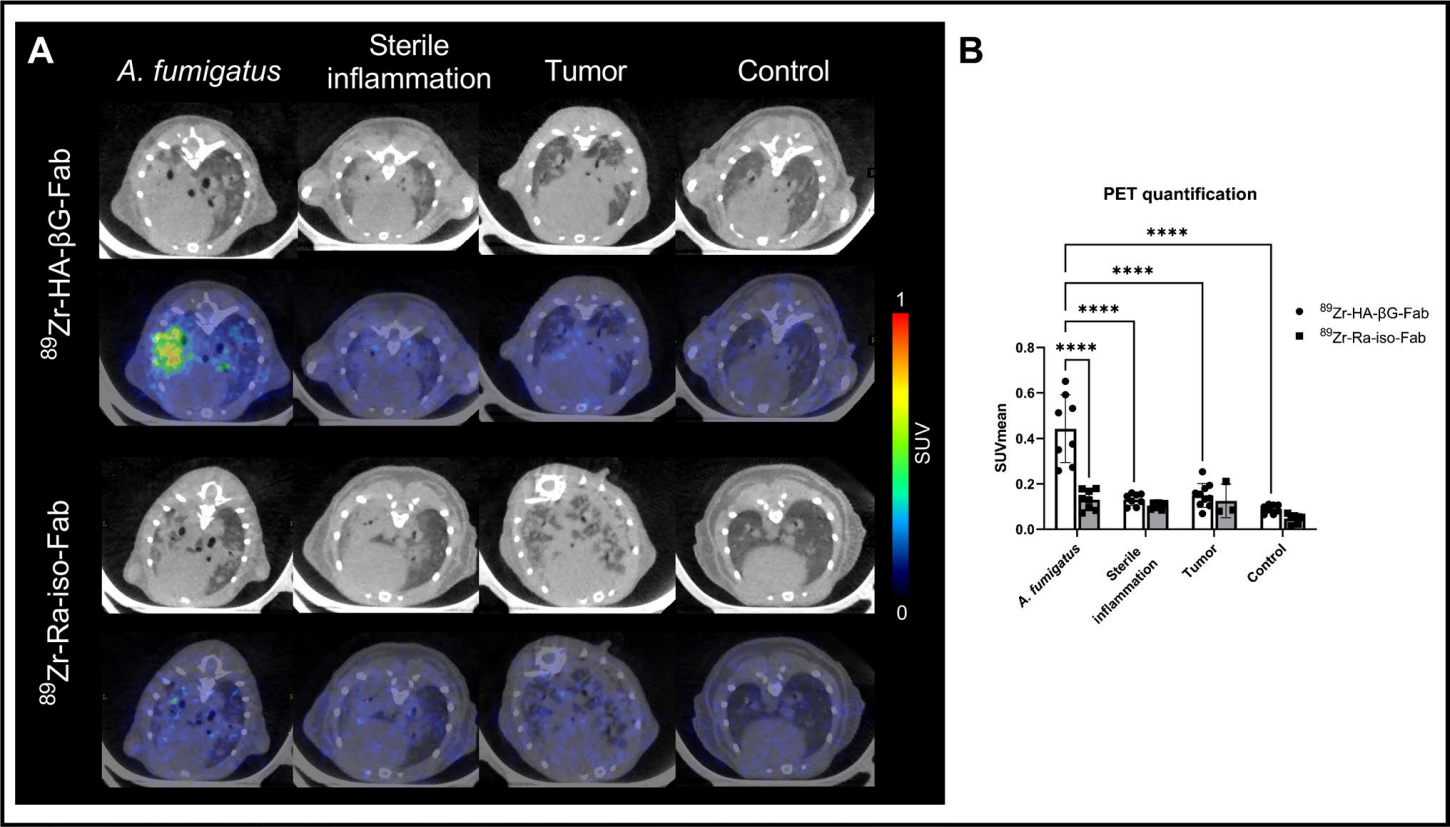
Static PET/CT imaging of *A. fumigatus* lung infection and inflammation models with ^89^Zr-HA-βG-Fab and ^89^Zr-Ra-iso-Fab. A Representative axial images of lung CT and PET/CT fusion are shown from *A. fumigatus* pulmonary infection (column 1), poly (I:C)-induced sterile lung inflammation (column 2), lung tumors (column 3) and healthy controls (column 4), 24 h after tracer injection. B PET quantification of binding in the lung consolidations (*n* = 7–10, except for tumor model injected with.^89^Zr-Ra-iso-Fab: *n* = 3). Two-way ANOVA with multiple comparisons was used for statistical analysis. , *P* < 0.0001
